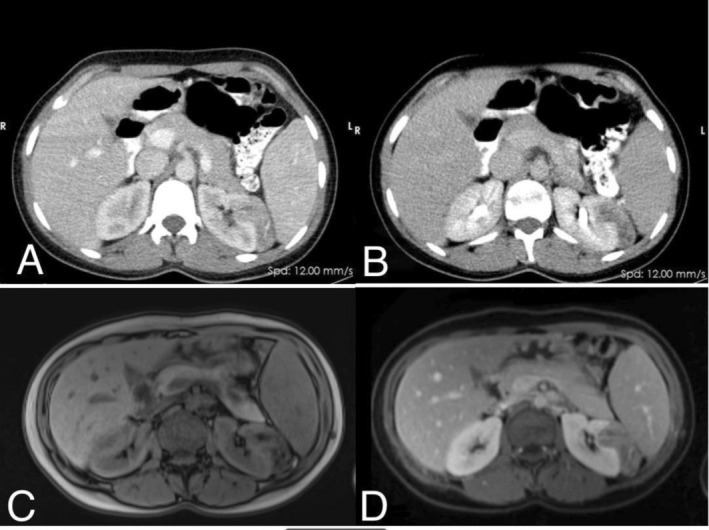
(A, B) Axial CT scan showing an irregularly shaped, hypodense mass with a heterogeneous pattern in the left kidney (A: Arterial phase, B: Delayed phase). (C, D) Axial T1‐weighted MRI demonstrating a lobulated, heterogeneous mass with fat content in the mi d‐pole of the left kidney.
